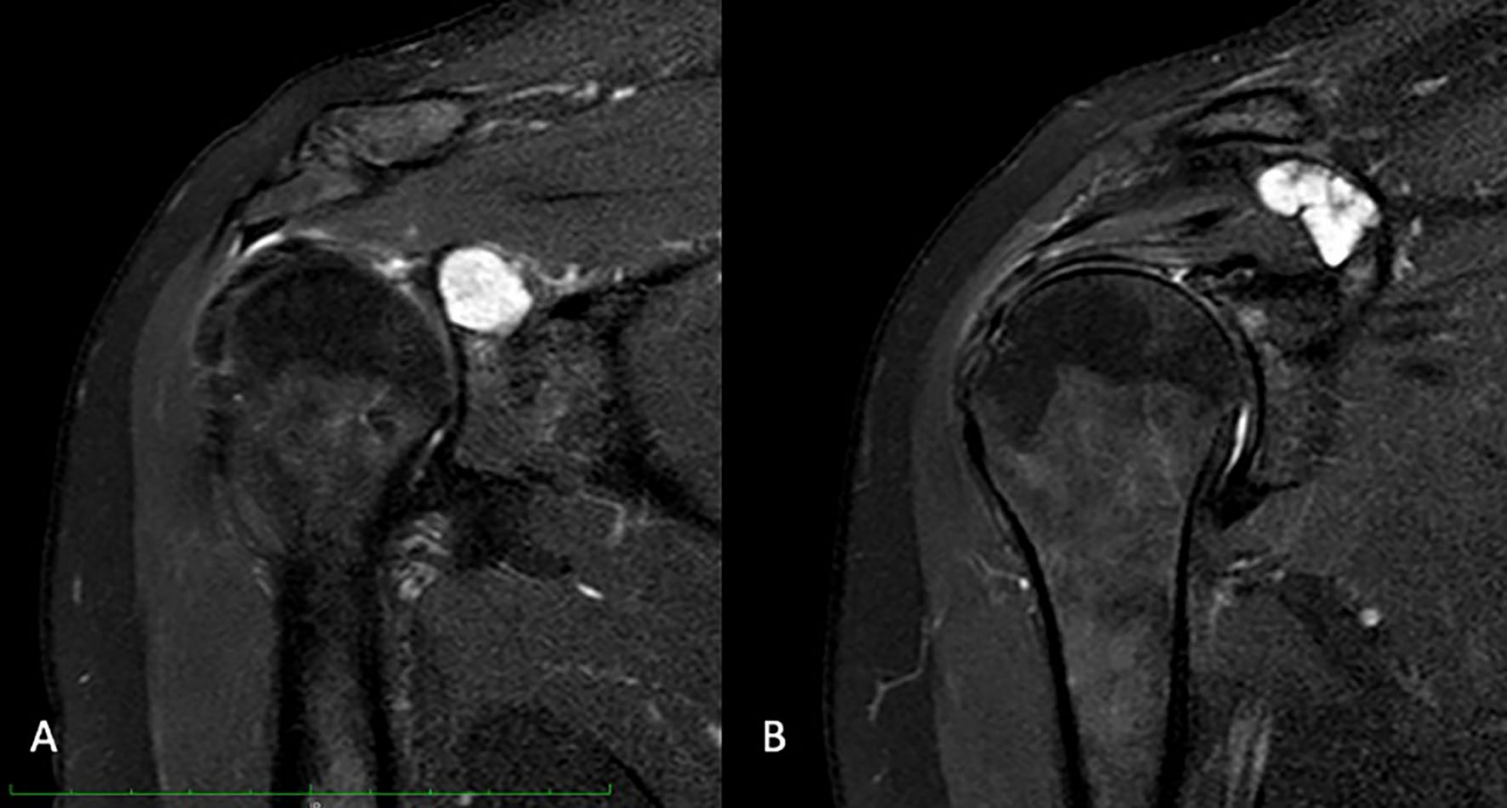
Preoperative T2 signal MRI of 56-year-old patient with a recurrent Grade 2 Chondrosarcoma of glenoid (A) and coracoid process (B)
